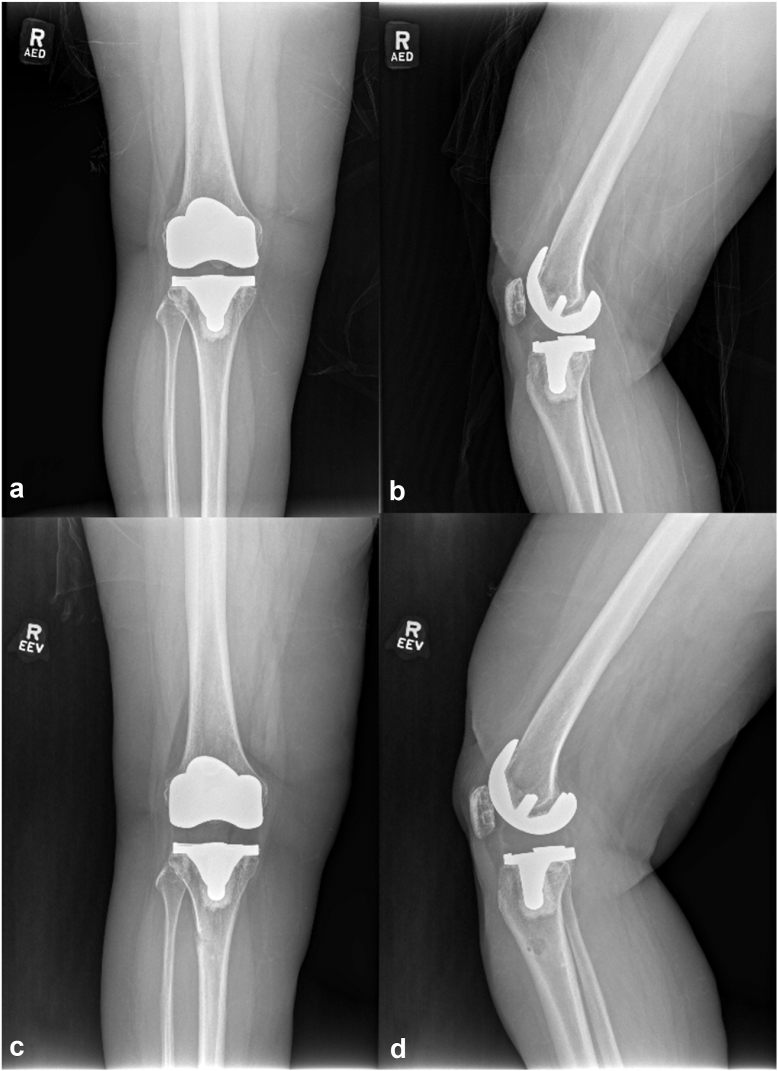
Demonstration of mid-flexion instability. (a and b) Preoperative anteroposterior and lateral radiographs of a 58-year-old female with a painful Microport Evolution pivot total knee system. Despite being well fixed and aligned, the knee exhibited mid-flexion instability. (c and d) Postoperative anteroposterior and lateral radiographs of the same patient. During surgery, the components were confirmed to be well fixed, and the polyethylene insert thickness was increased from a 12-mm cruciate-sacrificing insert to a 17-mm cruciate-sacrificing insert. This adjustment resulted in equal flexion and extension gaps. At 2 years postoperatively, the patient is pain-free with a range of motion from 0° to 125°.
